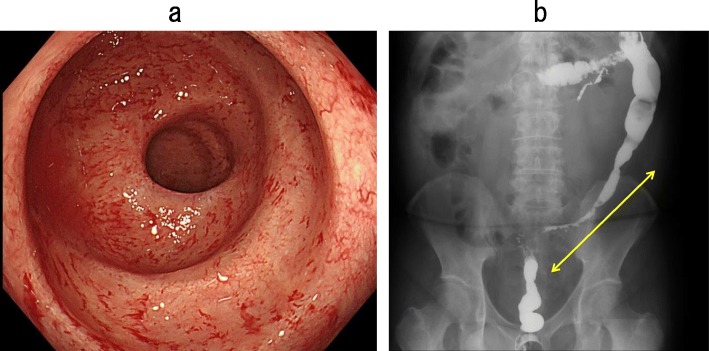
Colonoscopy 6 months after the surgery showed neither ulceration nor stenosis in the rectum (a), but an enema X-ray examination showed severe stenosis in the descending and sigmoid colon (b, yellow line)
